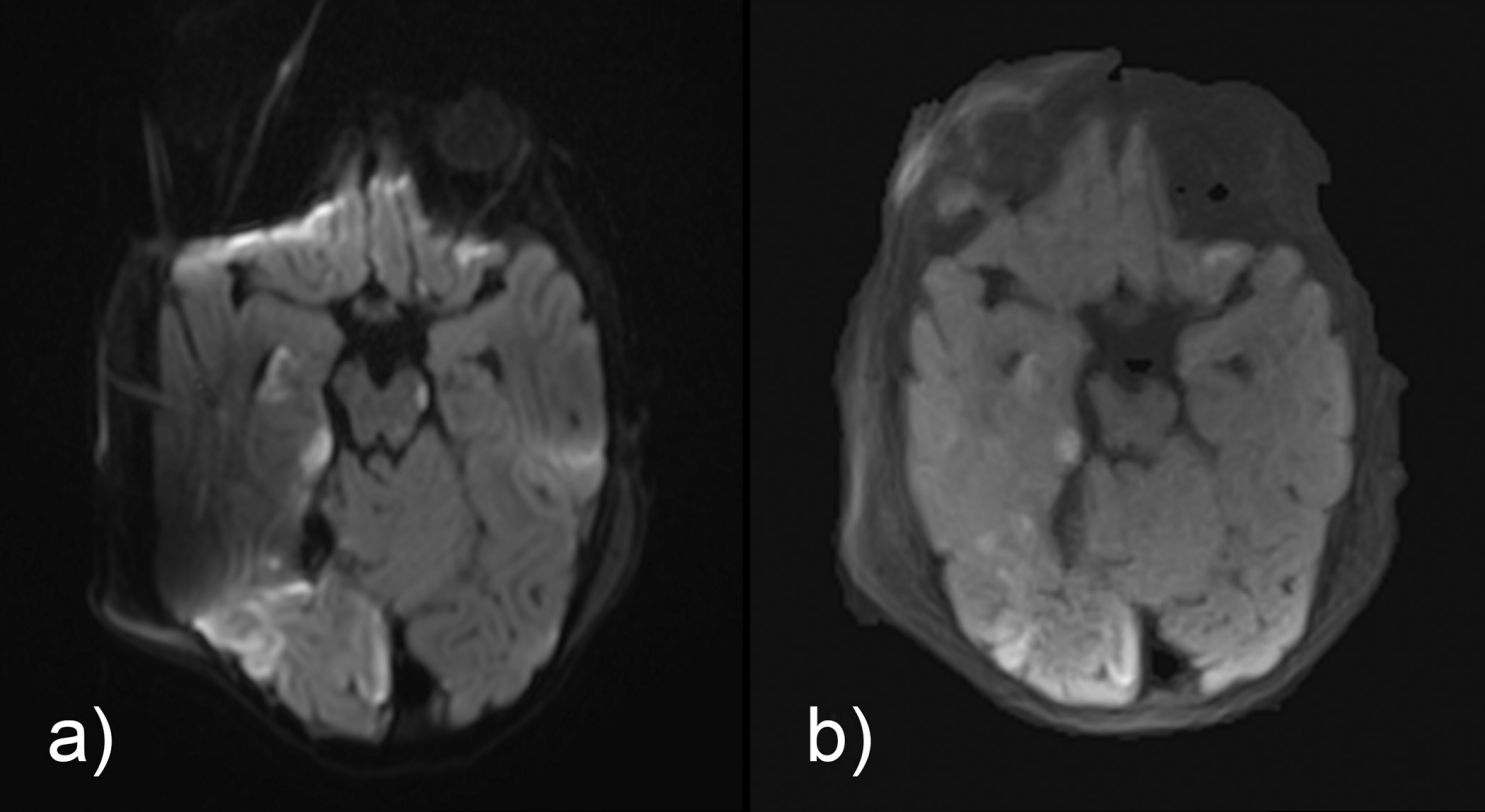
Comparison of isoDW images obtained by (a) EPI-DWI and (b) STEAM-DWI in a 14-month-old girl with posttraumatic epidural hemorrhage and partial infarction in the area of the right posterior cerebral artery (postoperative situs). Susceptibility effects due to the surgical foreign material on the calvaria and an intracranial pressure probe (in adjacent layer) result in moderate distortion and hyperintensity artifacts, which are not noticeable in STEAM-DWI. Please note that the hyperintense alterations in the EPI-DWI right frontal are exclusively due to artifacts. Depiction of the small hyperintense spot in the left crus cerebri in STEAM-DWI is inferior compared to EPI-DWI.
